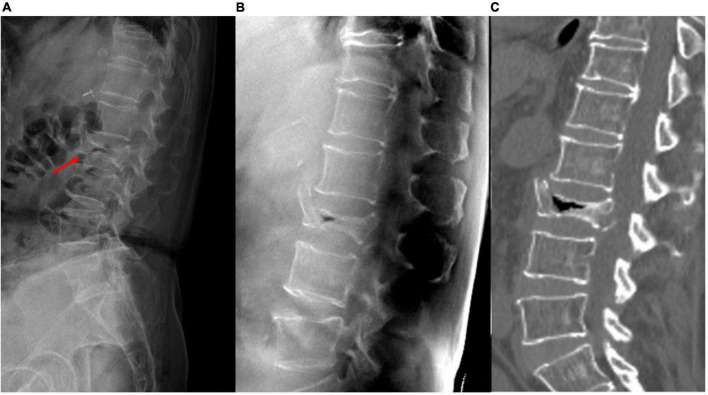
A patient with acute L2 vertebral compression fracture (VCF). (A) Plain radiograph shows bowel gas superimposed on the spine, and the fracture lines of the collapsed vertebra cannot be clearly seen. (B) Tomosynthesis and (C) CT both clearly show the extent of fractures and the air-filled cleft.
